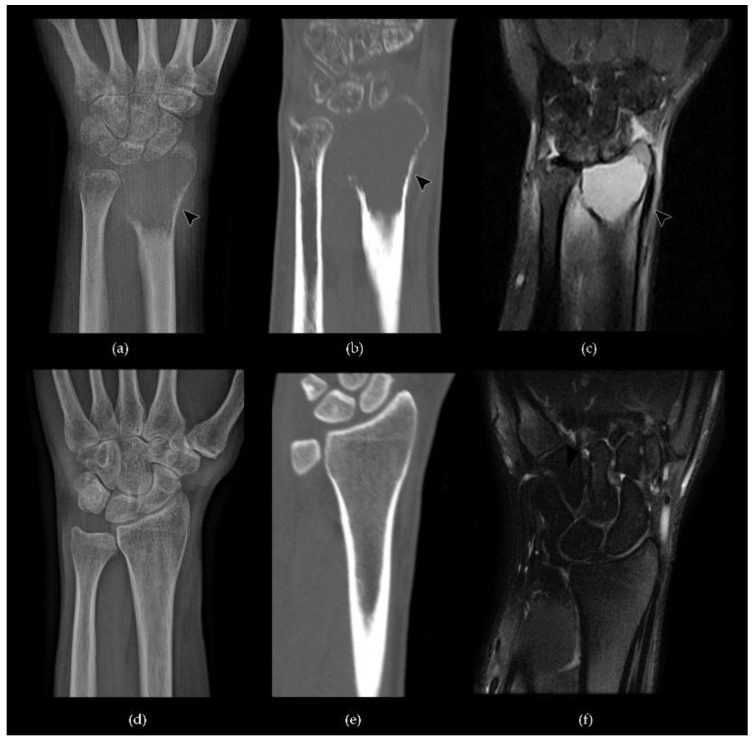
A case of GCTB of the distal metaepiphysis of the right radius with the involvement of the radiocarpal joint (a–c, arrowhead) compared to normal bone (d–f): (a) conventional radiography shows an osteolytic lesion with cortical thinning and endosteal scalloping with no periosteal reaction; (b) a coronal computed tomography (CT) scan highlights multiple cortical interruptions; (c) a coronal STIR magnetic resonance imaging (MRI) sequence shows a lesion with homogeneous signal hyperintensity; (d–f) conventional radiography, CT and STIR MR imaging of normal bone findings.
